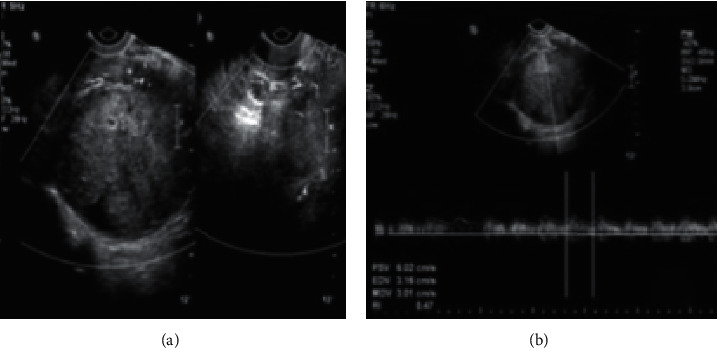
ADNEX combined with MRI in the same case for ultrasound ADNEX examination.
